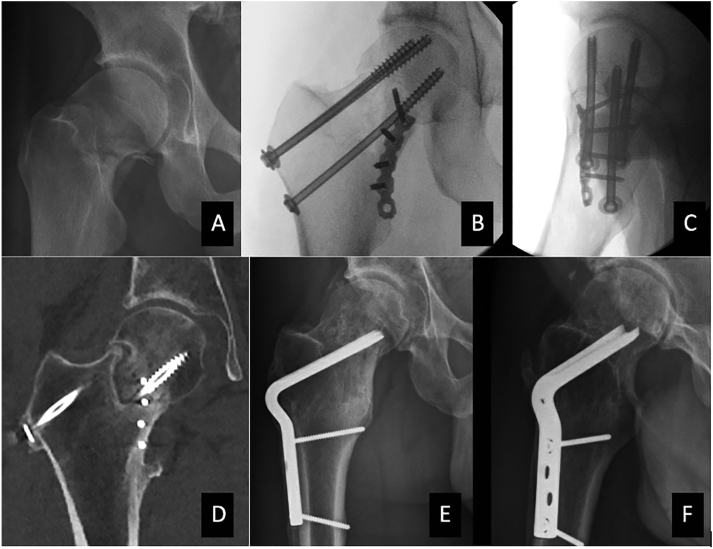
A: AO type 31B2.1 fracture of the right hip; B + C: Perioperative imaging of the anteromedial plate; D: CT scan that shows development of non-union 7 months after surgery; E + F: Complete healing 22 months after Pauwels osteotomy.
